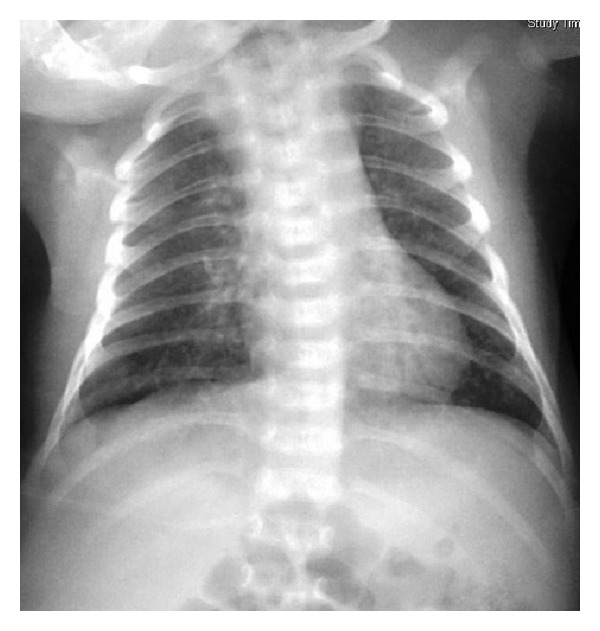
Diffuse patchiness on chest radiography in a neonate with *Chlamydia trachomatis*.
